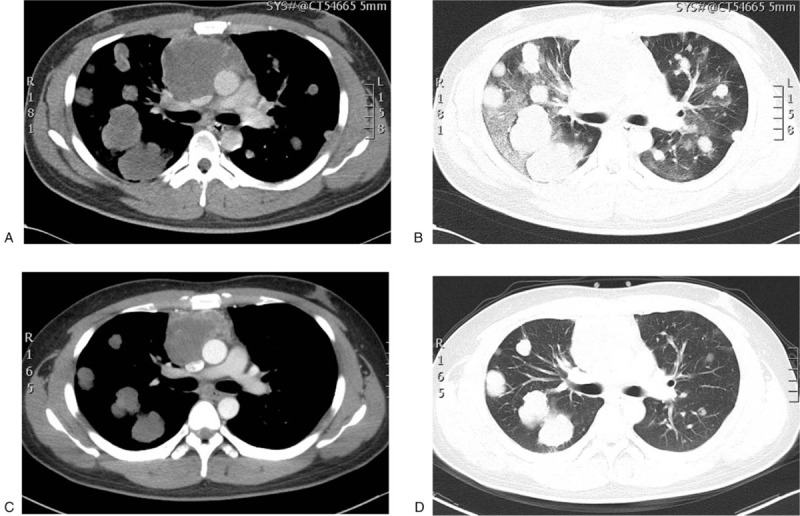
Chest CT scan shows a huge mediastinal mass and multiple bilateral pulmonary nodules prior to chemotherapy at the soft-tissue window (A) and the lung window (B). Both mediastinal and pulmonary lesions are partially reduced after four cycles of chemotherapy at the soft-tissue window (C) and the lung window (D).
